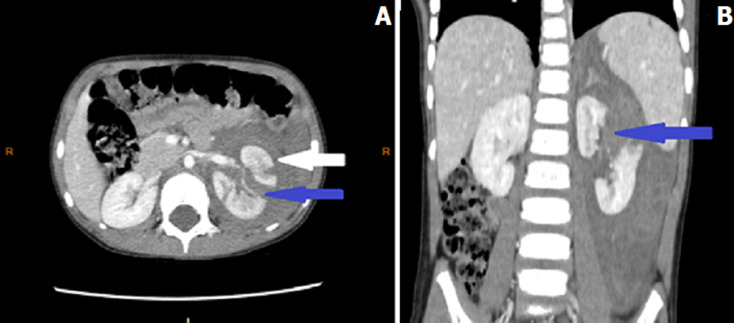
A) axial and B) coronal abdominal contrast enhanced CT performed after a blunt abdominal trauma in a 2-years-old boy showing an extended parenchymal laceration of the left kidney (grade IV of AAST Kidney Injury Scale) (blue arrows) associated with perirenal hematoma (white arrow) after falling from high and who was managed conservatively
